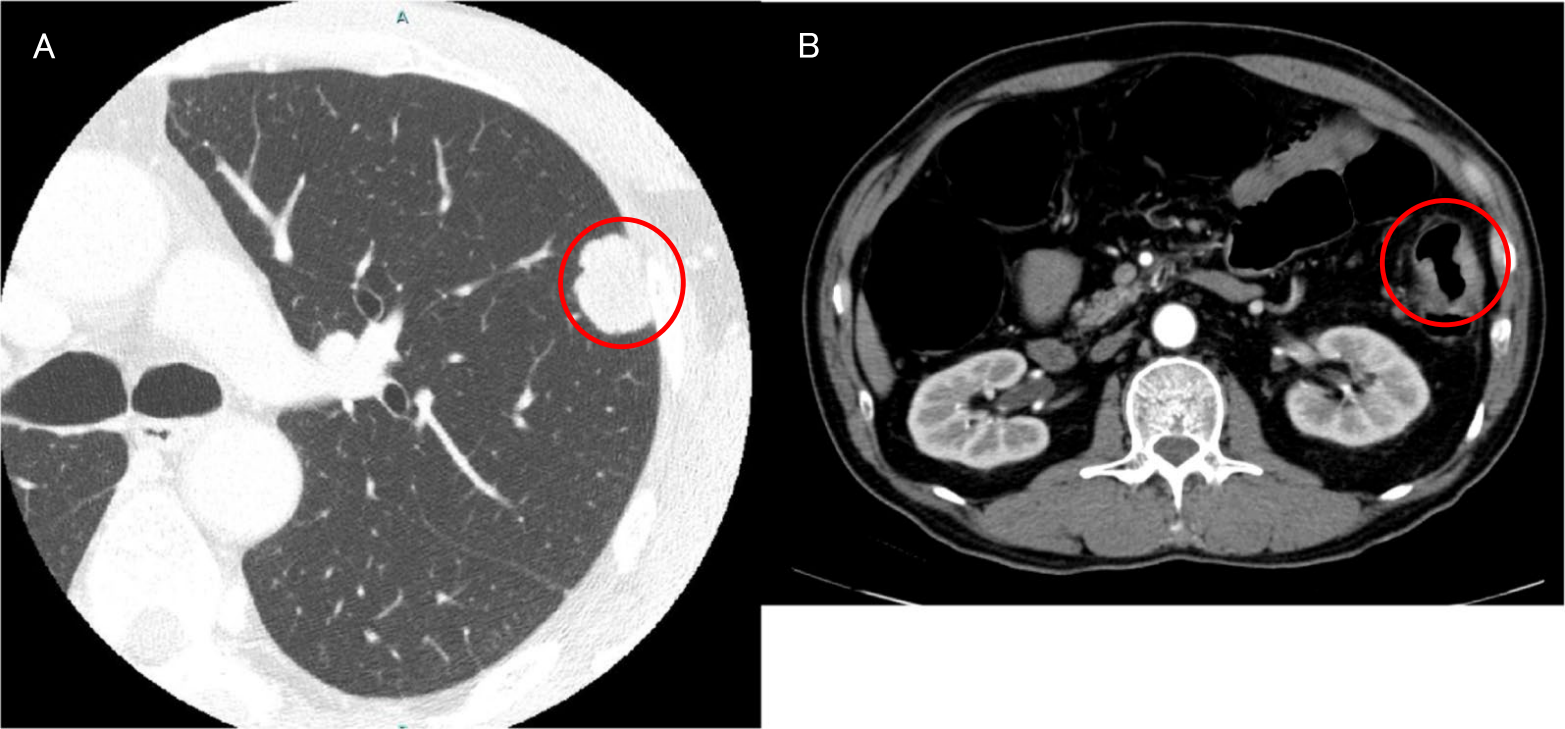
Computed tomography (CT) findings. A Plain chest CT revealed a solitary well-defined mass, measuring approximately 30 mm, in the superior region of the upper lobe of the left lung (red circle). B Abdominal contrast-enhanced CT revealed a subtotal mass with a contrast effect in the centre of the descending colon (red circle)
